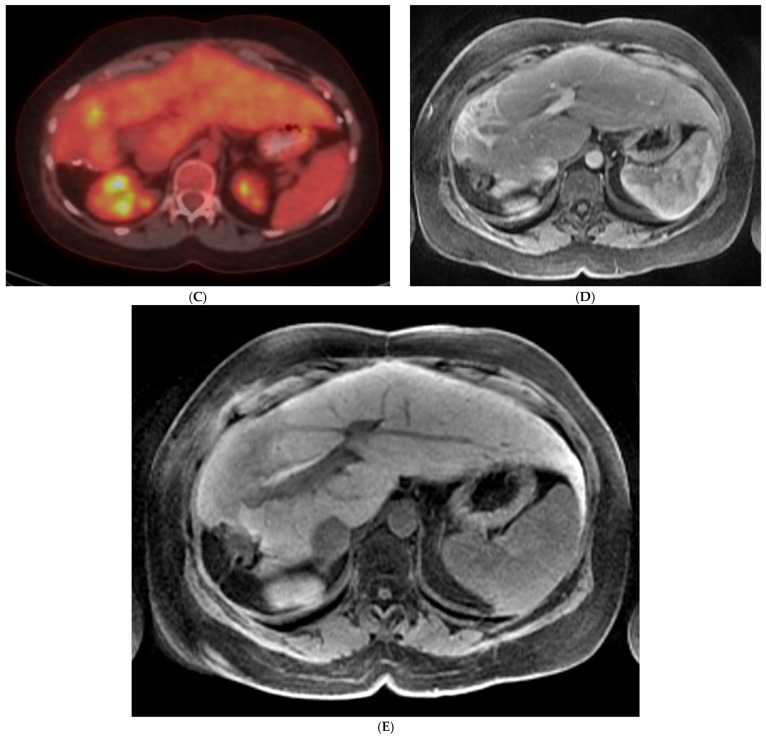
A 47-year-old woman with metastatic rectal cancer, status post chemotherapy, primary tumor, and posterior sector liver resection, with 2.9 cm segment 5 liver metastasis, as seen on the hepatobiliary phase of baseline MRI ((A); white arrowhead). This was treated with SBRT (6000 cGy in 5 fractions). PET/CT after 4 months showed persistent uptake in the treatment zone ((B); SUVmax 6.1; liver SUVmean 2.8). After 9 months, there was a continued decrease in FDG uptake in the treatment zone, above that of background liver ((C); SUVmax 3.4; liver SUVmean 2.4). MRI on the same day showed geographic area of arterial enhancement (D), with decreased signal on the hepatobiliary phase (E).
